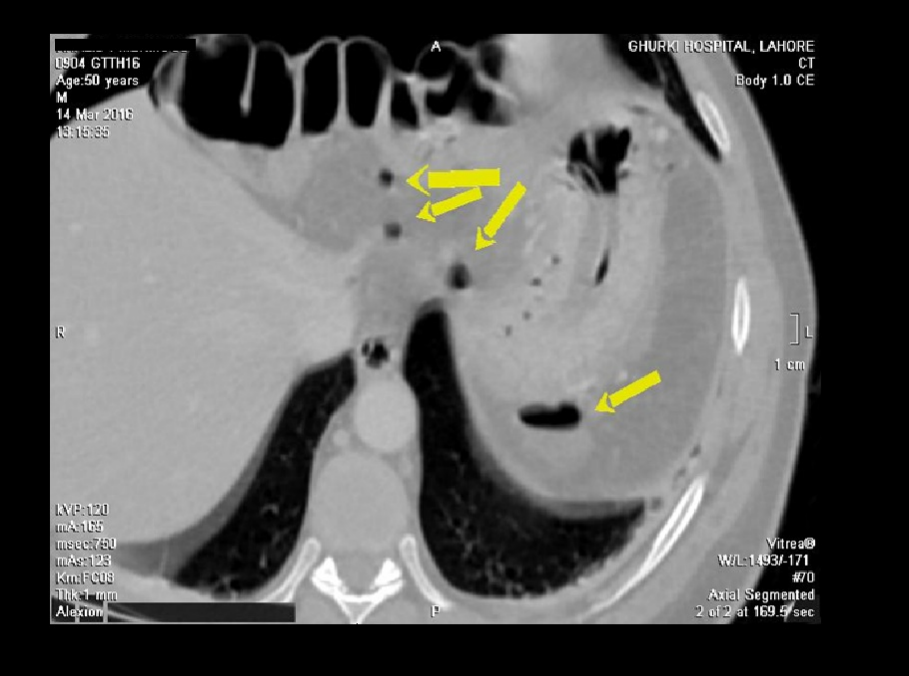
CT scan of the abdomen (axial segment): pneumo-peritoneum and a few air pockets (yellow arrows) are appreciated around the stomach, which may be most likely a result of gastric perforation.
